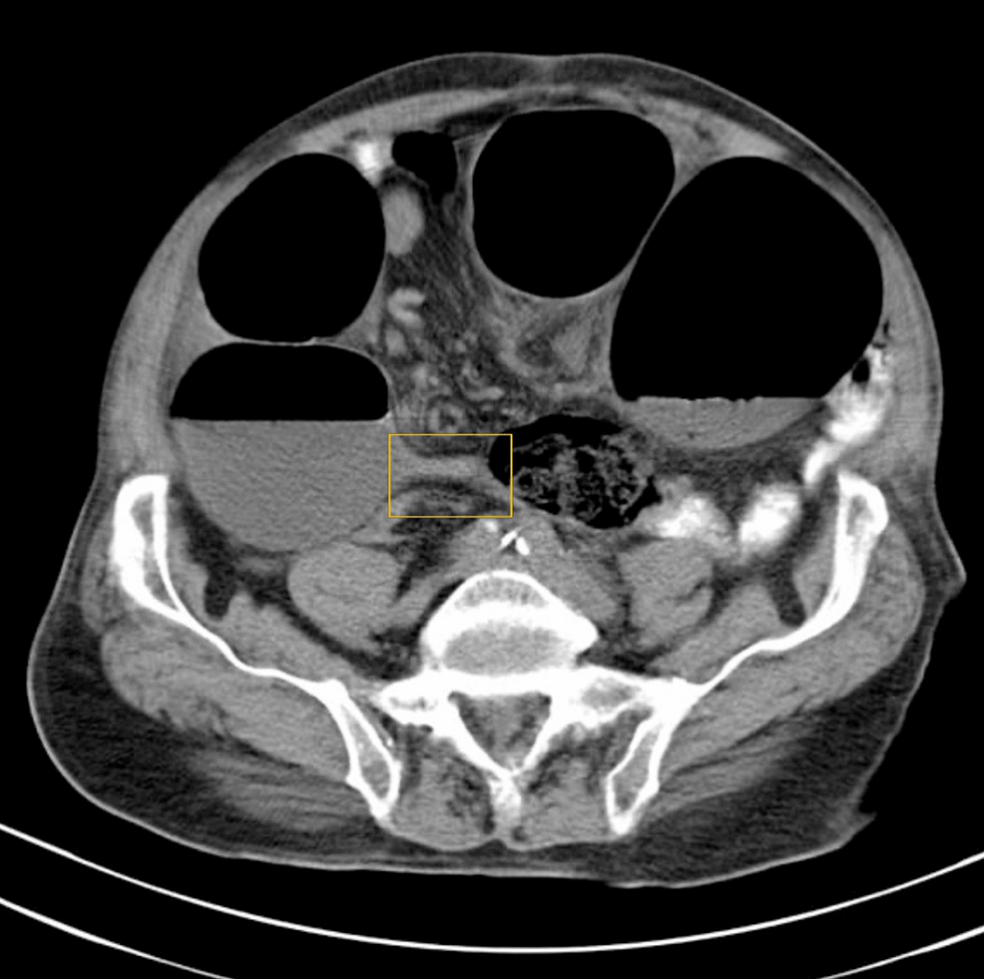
Computed tomography image of the patient The highlighted area indicates the transition point of the sigmoid volvulus.
